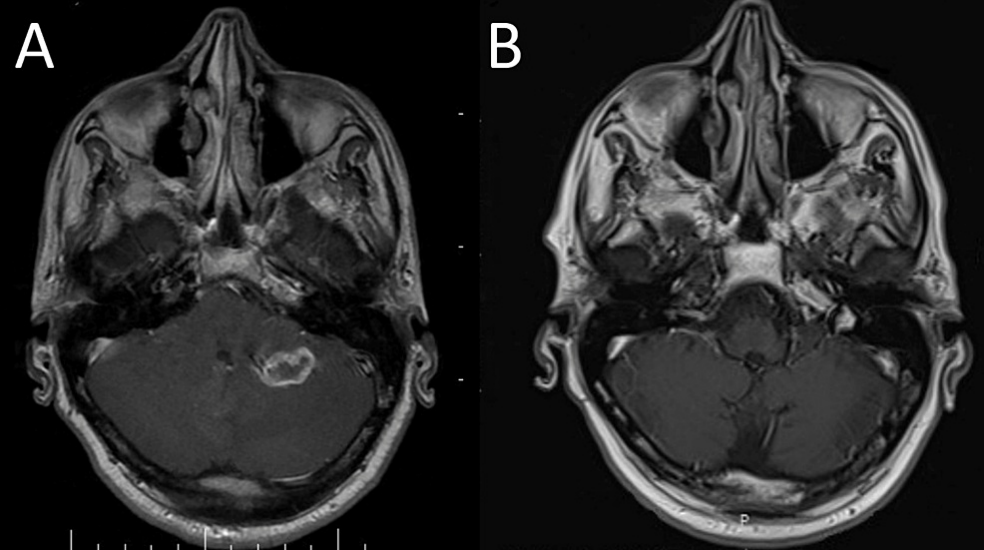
Pre-treatment (A) and post-treatment (B) brain MRI Pre-treatment (A) and post-treatment (B) brain MRI showing complete resolution of metastatic disease in the posterior fossa following the combined treatment with RT and nivolumab (28 months apart).
